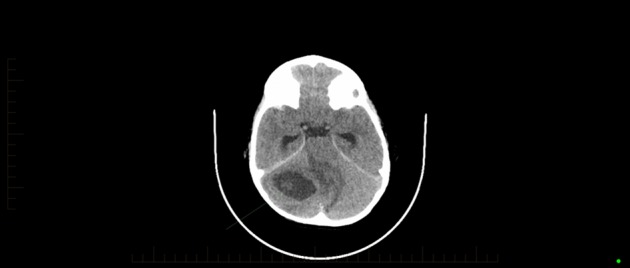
Initial CT imaging demonstrating a cystic mass in the right cerebellar hemisphere with surrounding oedema and mass effect.
